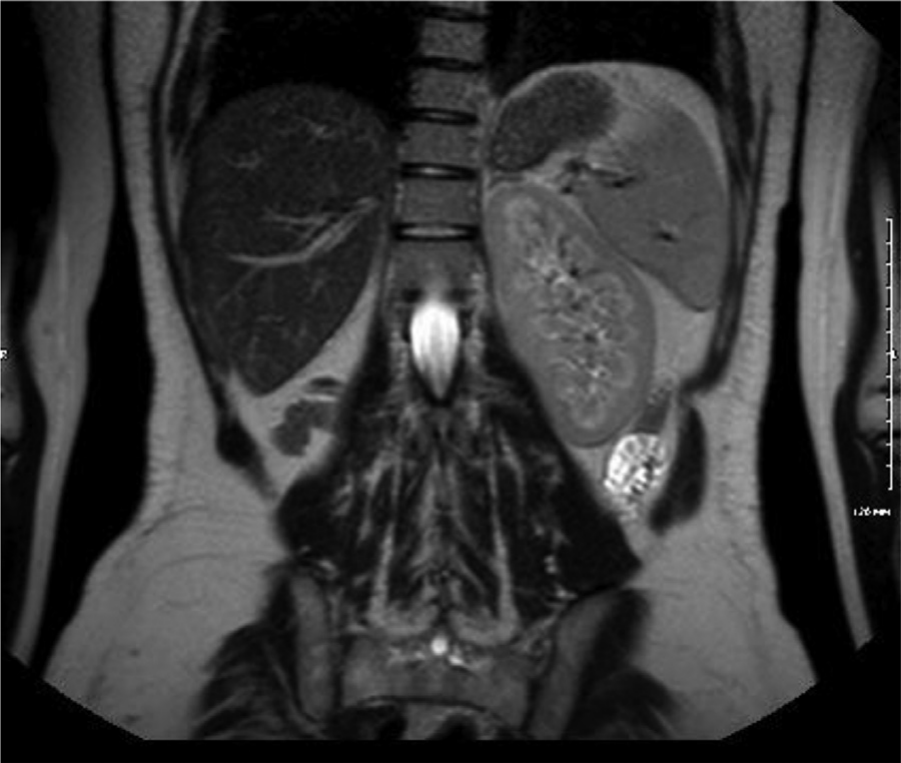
MRI T2-Weighted coronal. Right kidney is not visualized. There is mild compensatory hypertrophy of the left kidney in the setting of right renal agenesis.
